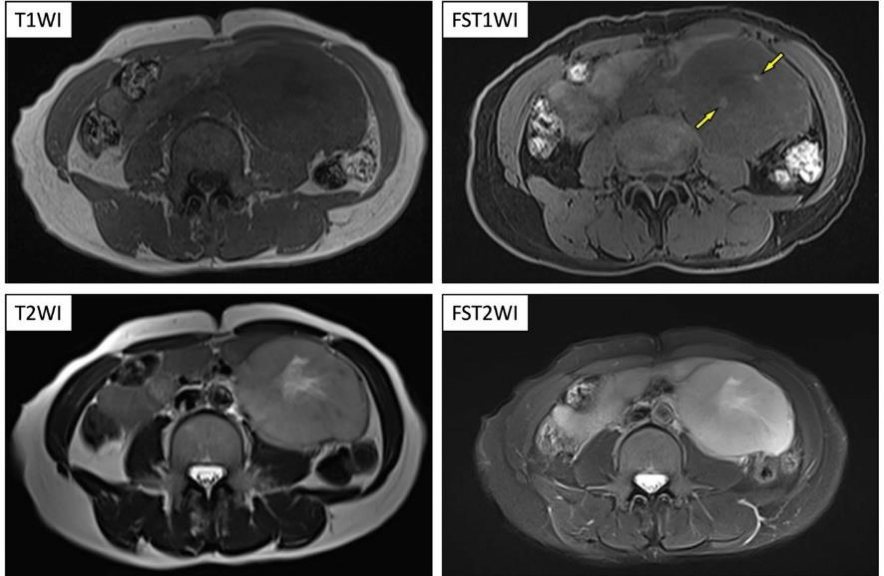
Magnetic resonance imaging showing low-signal lesions on T1-weighted imaging (T1WI) and high-signal lesions on T2-weighted imaging (T2WI). A signal change in the center of the lesion suggested degeneration or necrosis can be observed. Fat-suppressed T1WI (FST1WI) revealing a faint high-signal area within the lesion (⇨)—a finding suspicious for internal haemorrhage. FST2WI, fat-suppressed T2WI
